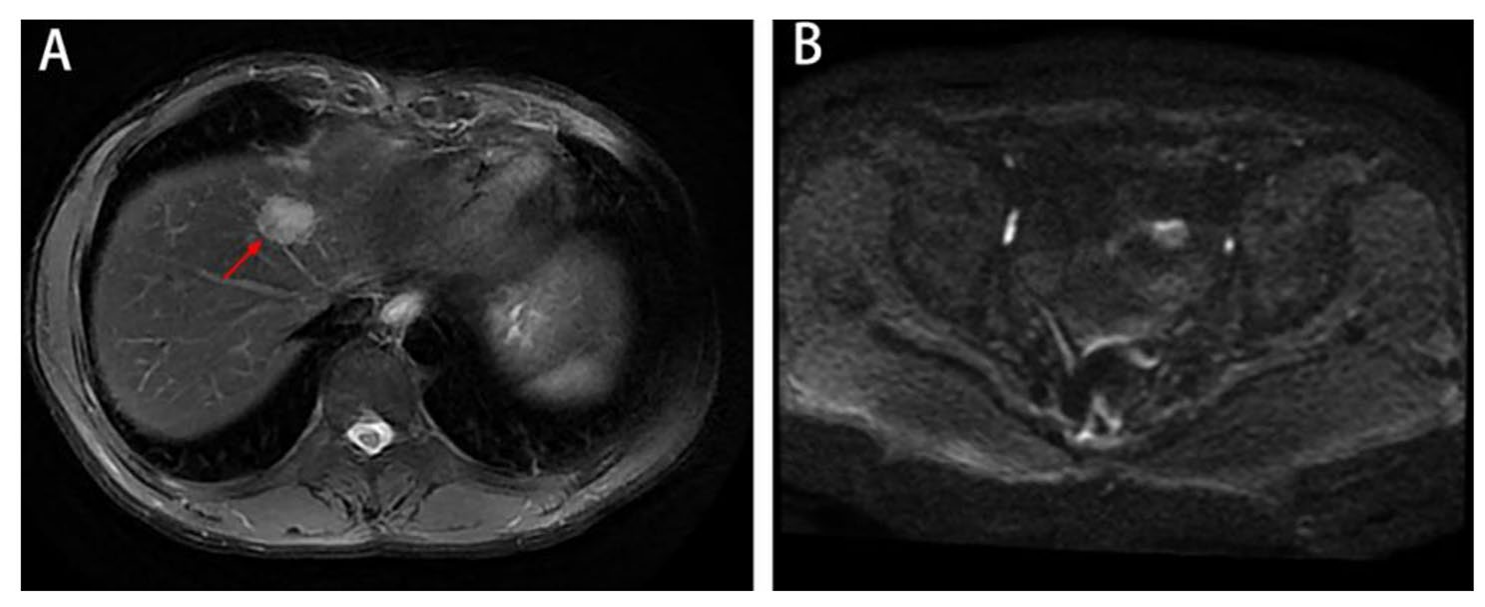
Imaging data from January 2022 A-B: MRI revealed a single metastatic lesion (2.5 cm) in the fourth segment of the liver, with no lesions in the pelvis
